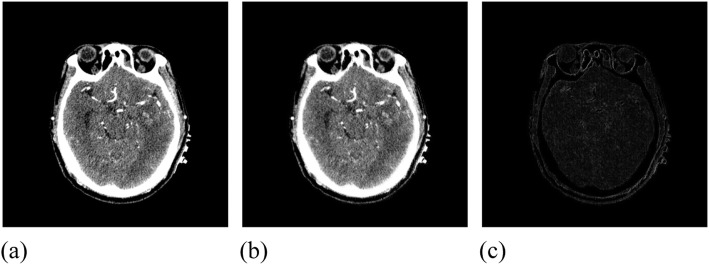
Initial image data. (a): CT reference image; (b): CT moving image; (c): Difference between CT reference image and moving image
